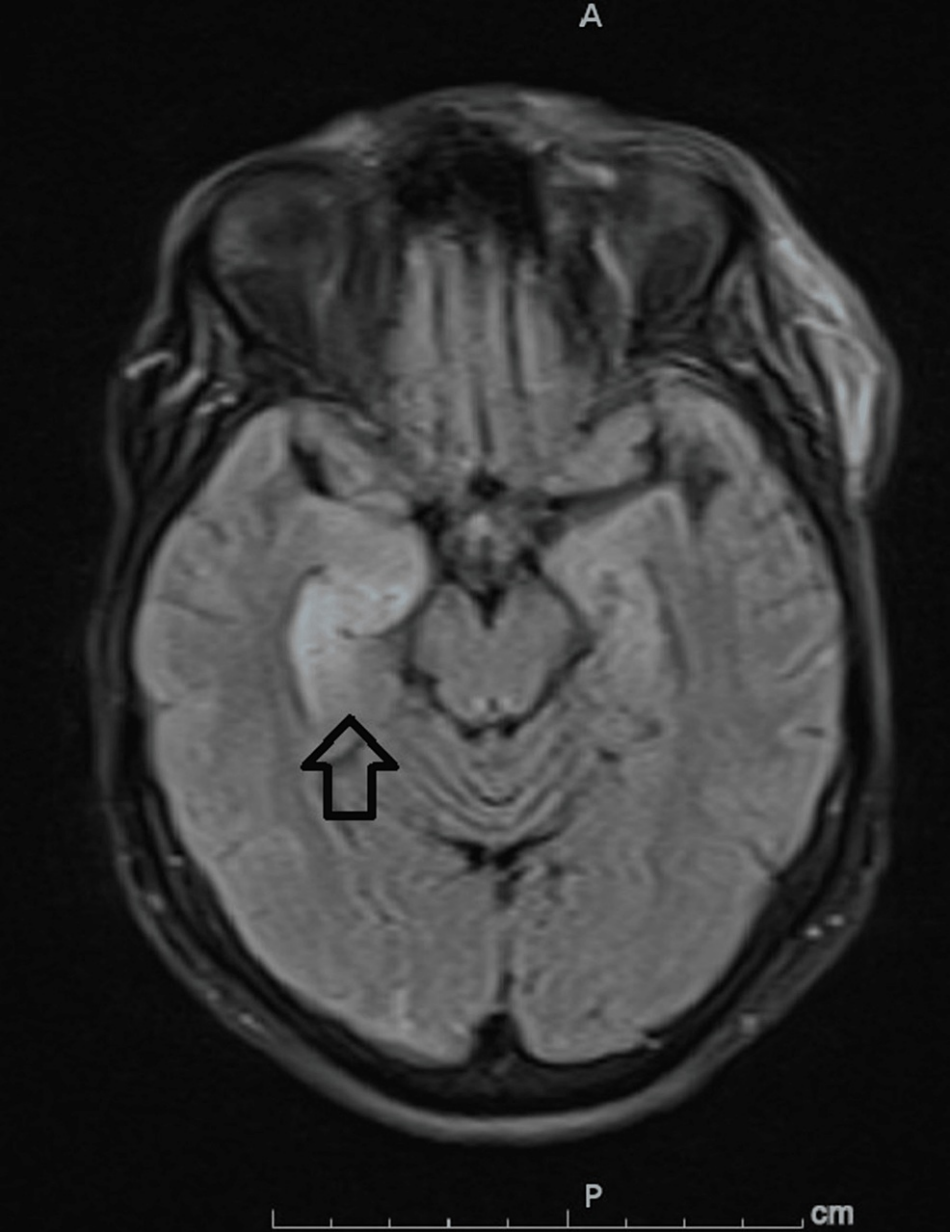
MRI Brain FLAIR MRI brain showing right-sided temporal lobe hyperintensity (arrow).
